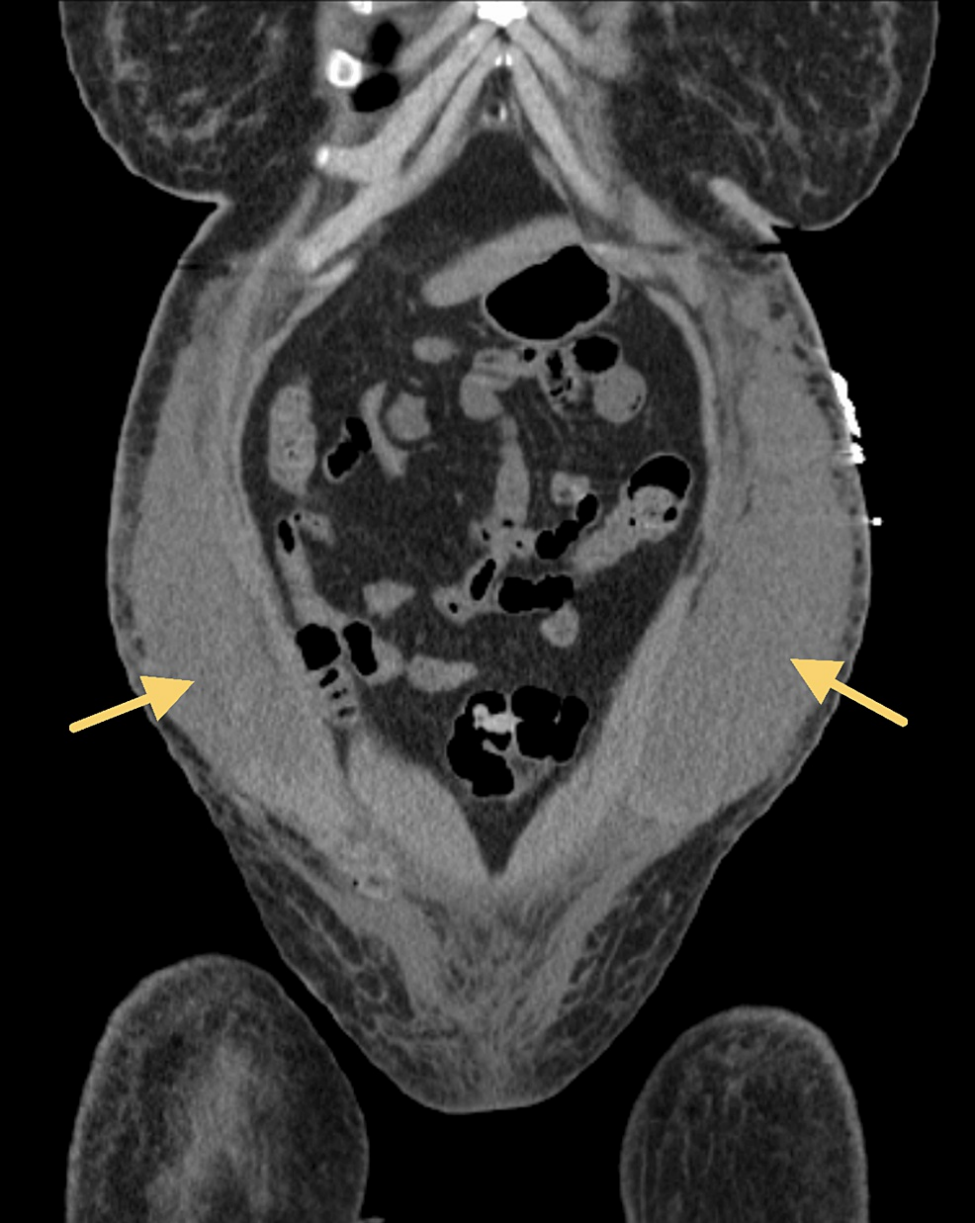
Computed tomography coronal view of hematoma, intra-abdominal and pelvic free fluid in abdominal cavity bilaterally (arrows).
